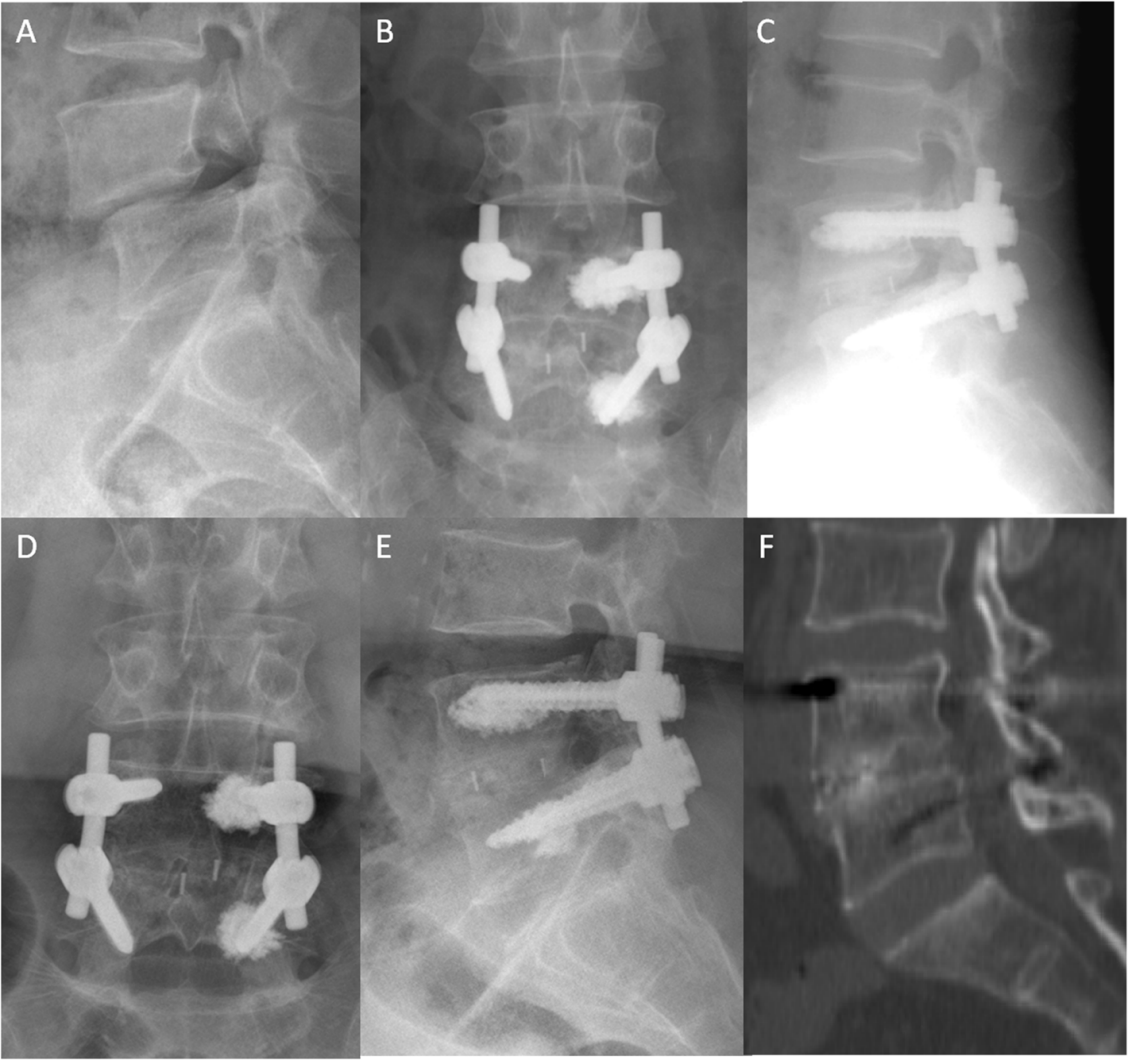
A 65-year-old female diagnosed with spondylolysis at the L4 vertebral body with osteoporosis (*T* = −3.2). a Preoperative lateral X-ray showing grade I lumbar spondylolisthesis. b–c Unilateral PMMA-augmented CPSs are used for spinal fixation. Immediate postoperative radiographs showing reconstruction for spondylolisthesis without PMMA leakage. d–e CPSs are observed in place after 49 months of surgery. f CT scan showing that bony fusion was achieved
